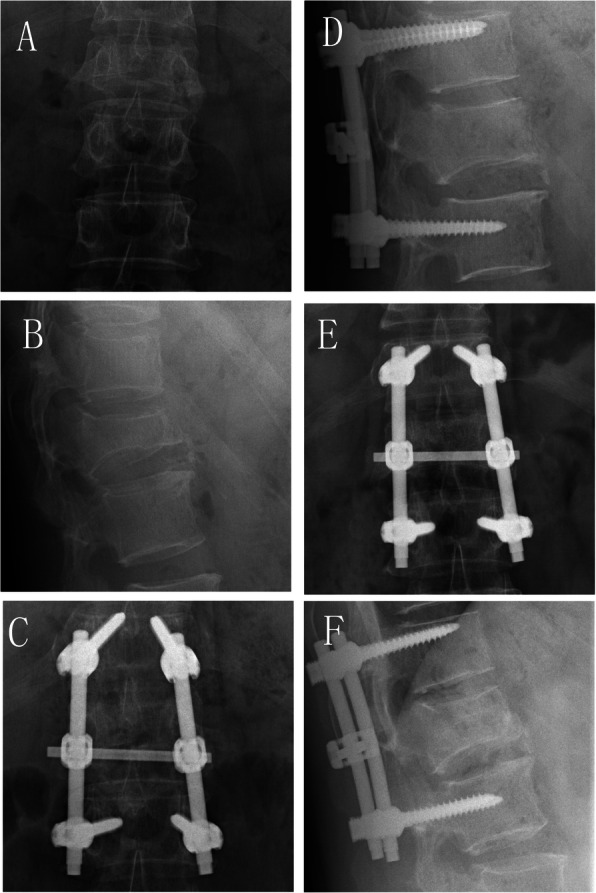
Imaging of a typical patient who underwent short-segment transpedicular screw fixation alone before and after the operation. A, B: Preoperative coronal and sagittal x-ray film demonstrating a burst fracture of the vertebral body. C, D: Coronal and sagittal x-ray film 3 days after the operation. E, F: Coronal and sagittal x-ray film 12 months after the operation
